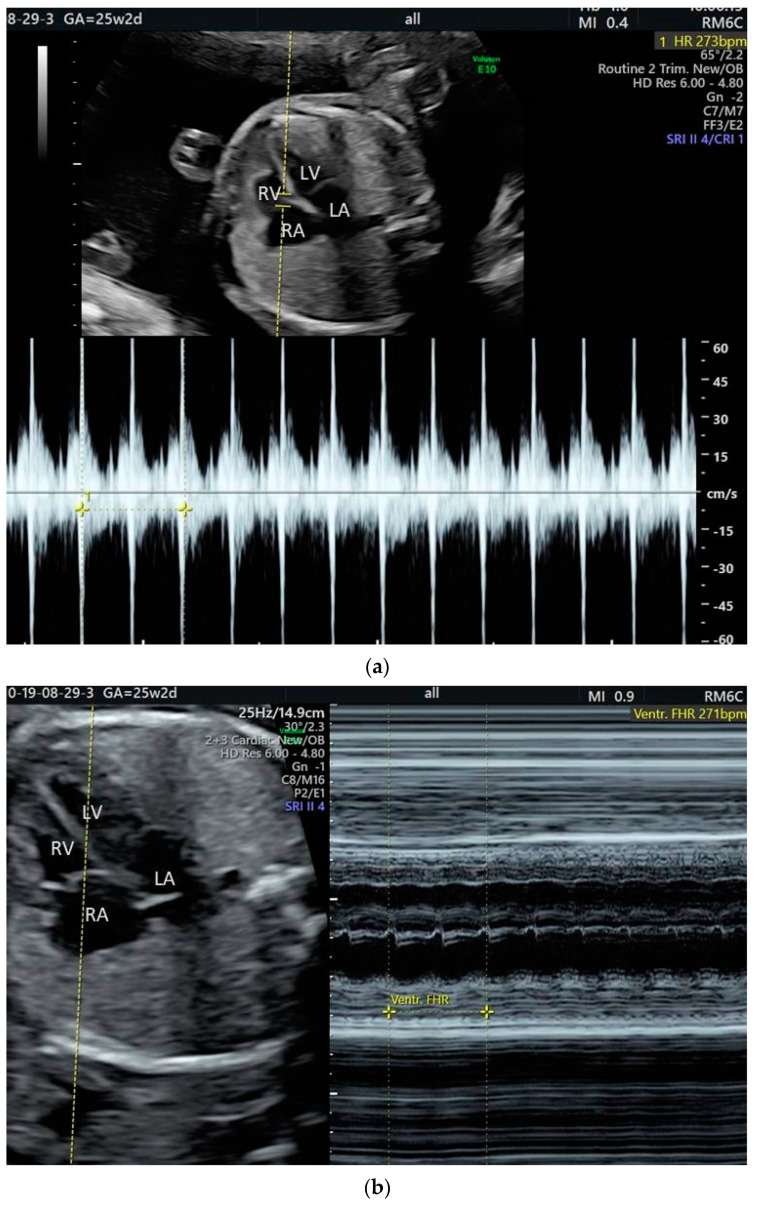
Fetal supraventricular tachycardia: (a) Pulsed Doppler analysis; (b) M-mode analysis.
